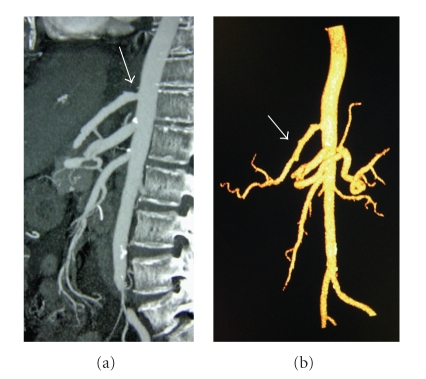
(a) Thin section two-dimensional sagittal CTA reconstruction of the aorta shows the anastomosis (arrow) of the hepatic artery to the proximal abdominal aorta superior to the celiac trunk and superior mesenteric artery (SMA). (b) Colorized three-dimensional volume rendered obliquely oriented CTA reconstruction shows the course of the donor hepatic artery (arrow) from the aorta to the proper hepatic artery bifurcation.
